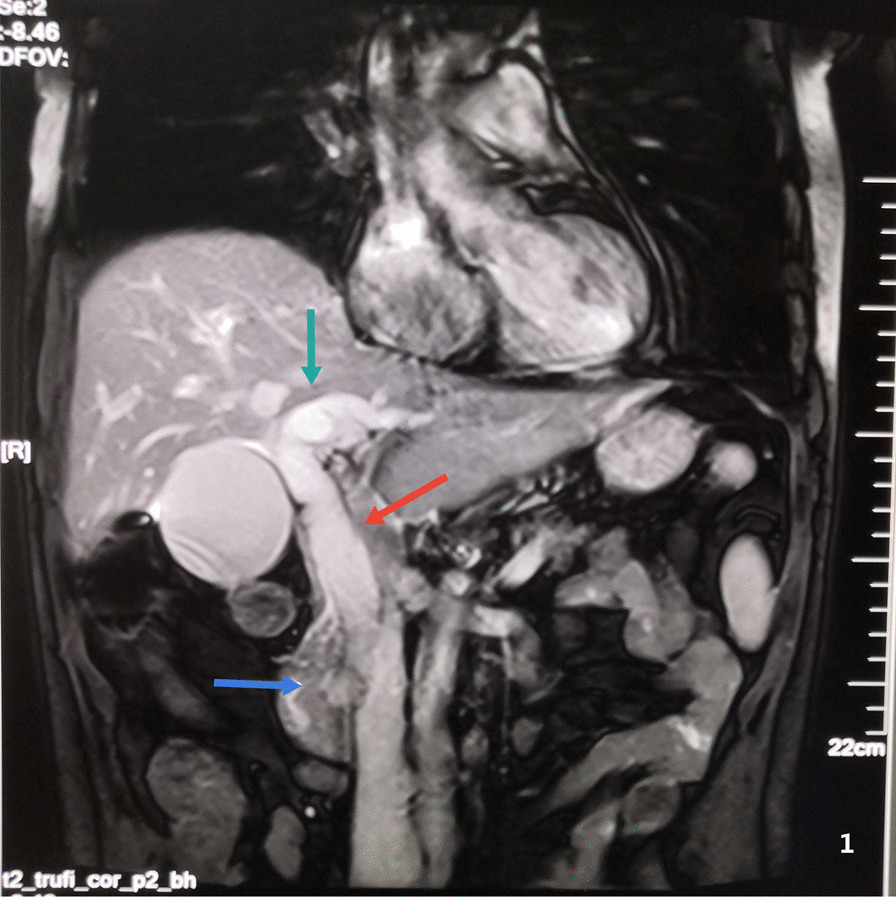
Abdominal MRI. Blue arrow: neoplastic lesions of the duodenal papilla. Green and red arrows: severe dilatation of intrahepatic and extrahepatic bile ducts
